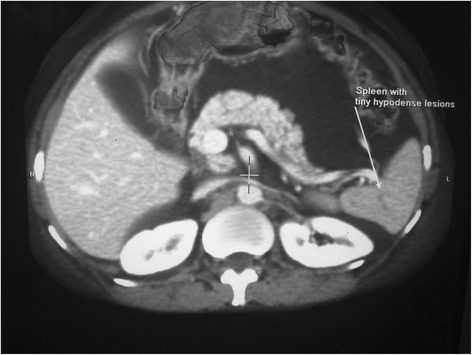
Contrast-enhanced computed tomography scan of abdomen shows splenic lymphangiectasia.
